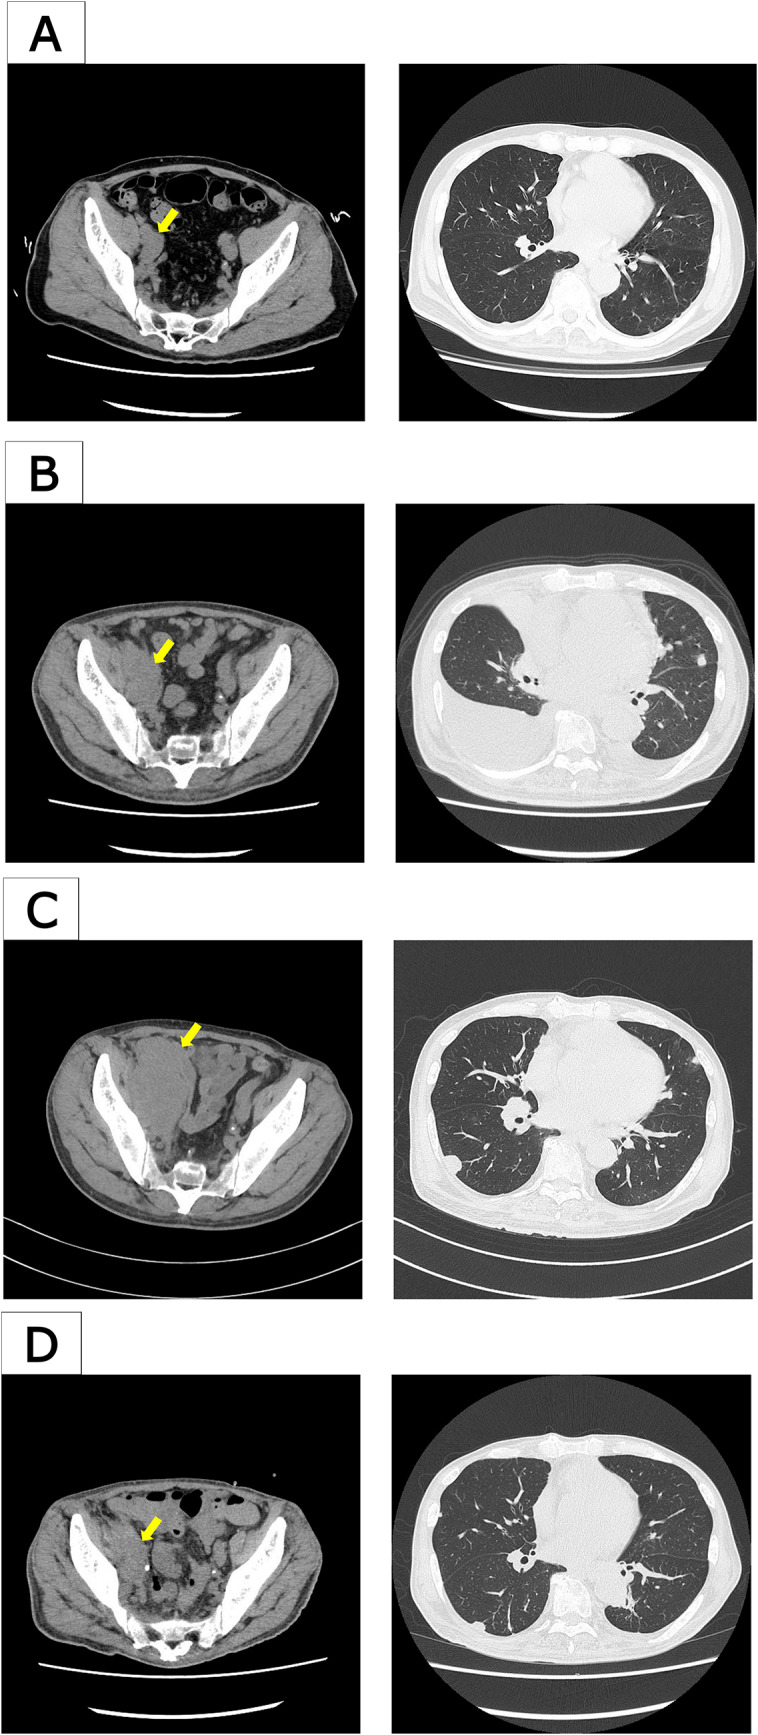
CT images before and after treatment modification. A Enlargement of the right external iliac lymph node without pulmonary metastases before initiation of triplet therapy. B Right external iliac lymph-node metastasis and appearance of pulmonary metastases after starting triplet therapy but before docetaxel administration. C After completion of three cycles of docetaxel (end of triplet therapy), showing progression with enlargement of the same lymph-node and new pulmonary metastases. D After two cycles of carboplatin plus etoposide (CBDCA + VP-16), partial regression of the lymph nodes and pulmonary lesions was observed
